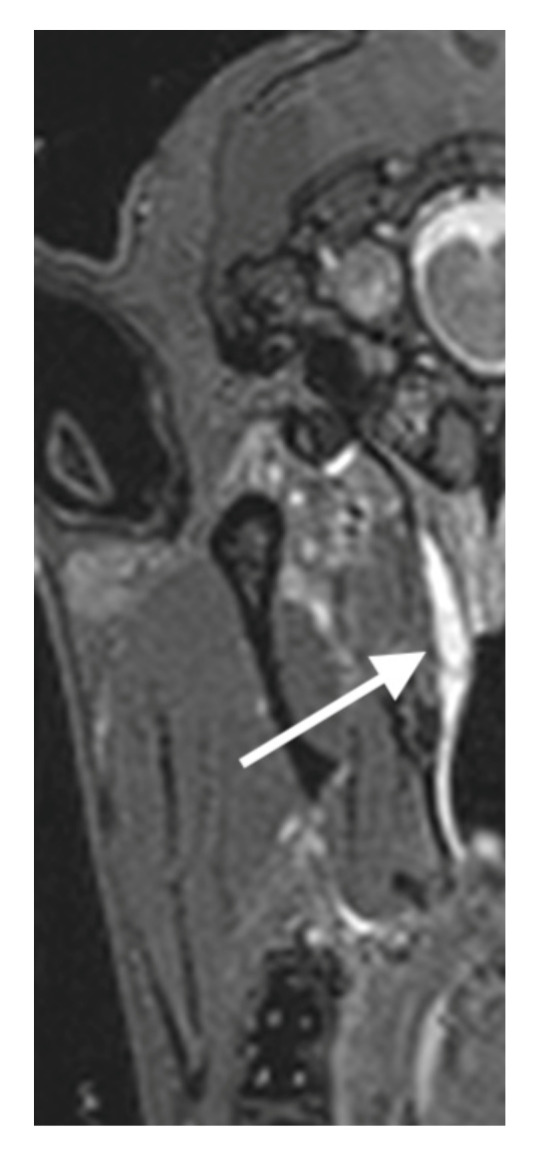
MRI image of case 21 after 13 weeks. The HA depot is marked by a white arrow.
